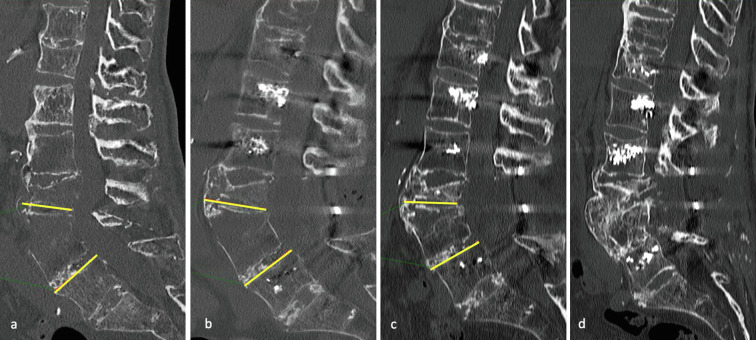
84-year-old female with a hyperextension fracture of L4, treated by open posterior cemented osteosynthesis (radicular decompression associated), with 47° of anterior diastasis in the pre-operative CT scan (a). Immediate post-operative CT scan showing no relevant reduction of the anterior diastasis measured at 43° (b). At 6 months, the CT scan shows a significant reduction to a 30° anterior diastasis (c), allowing for consolidation through anterolateral osseous bridging (d).
